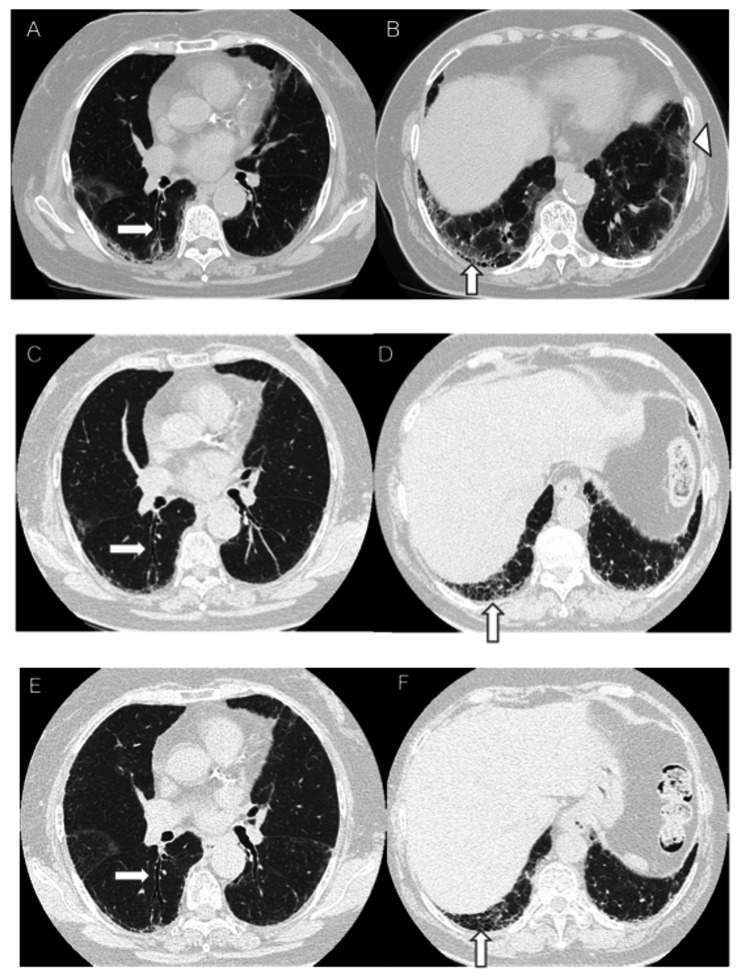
Representative longitudinal chest CT findings in an 84-year-old woman after hospitalization for COVID-19 (A, B) CT images obtained at hospitalization show extensive ground-glass opacity as the predominant pattern, with traction bronchiectasis (A, arrow) and honeycombing (B, arrow). The CT severity score (CTSS) was 19. (C, D) At three months after hospitalization, the predominant pattern had changed from ground-glass opacity to reticulation. Traction bronchiectasis (C, arrow) and honeycombing (D, arrow) remained visible. The CTSS decreased to 14. (E, F) At 12 months after hospitalization, reticulation remained the predominant pattern, with persistent traction bronchiectasis (E, arrow) and honeycombing (F, arrow). The CTSS remained 14.
